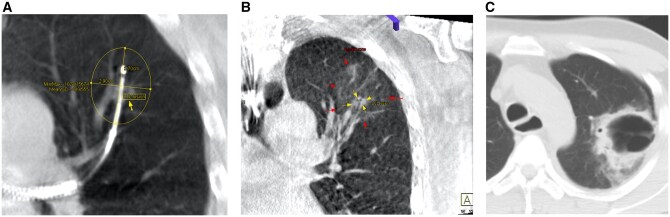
Patient A intraoperative CBCT and postoperative CT. (A) Intraoperative CBCT before TMWA showed a semi-solid cavitatory oligometastases and an optimal ablation catheter placement, with measurement of predicted ablation zone. (B) Intraoperative CBCT performed after ablation showed an adequate ablation zone covering the lesion with ample margins and no pleural complications. (C) Postoperative CT of the thorax was performed in view of the new onset of pneumothorax. CT showing an extensive ablation change with a large cavitation and a small amount of pleural effusion. A small fistula was noted at the periphery. Abbreviations: CBCT, cone-beam CT; TMWA, transbronchial microwave ablation.
